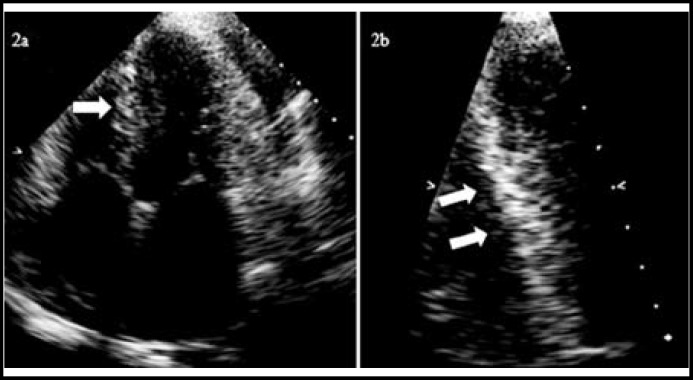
Echocardiography in a 57-year-old man with systemic amyloidosis. “Sparkling” appearance (white arrows) of ventricular septal wall showed in (a) four-chamber view and (b) ventricular septal wall view
